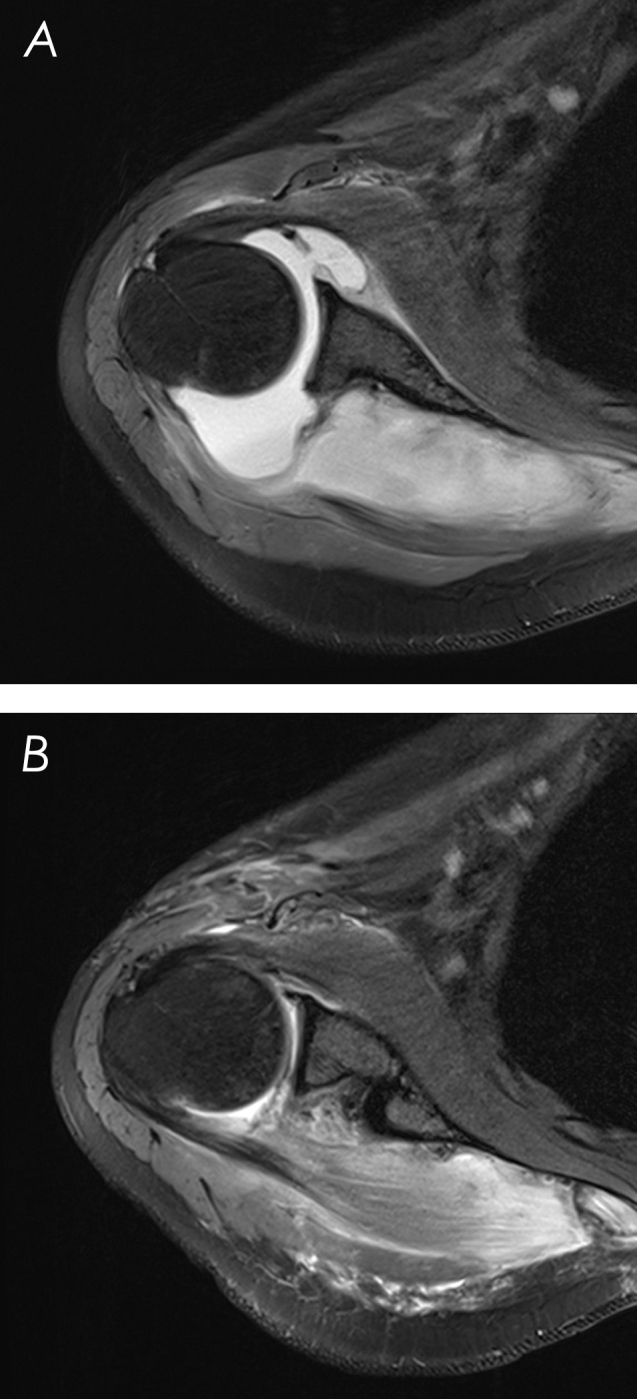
Shoulder MRI. (a) Preoperative, showing an extensive infraspinatus intramuscular abscess with extension to the supraspinatus, teres minor, and triceps medial head; significant glenoid joint effusion; and synovial enhancement and thickening. (b) Immediate postoperative, after abscess drainage and arthroscopic debridement of the glenohumeral joint.
